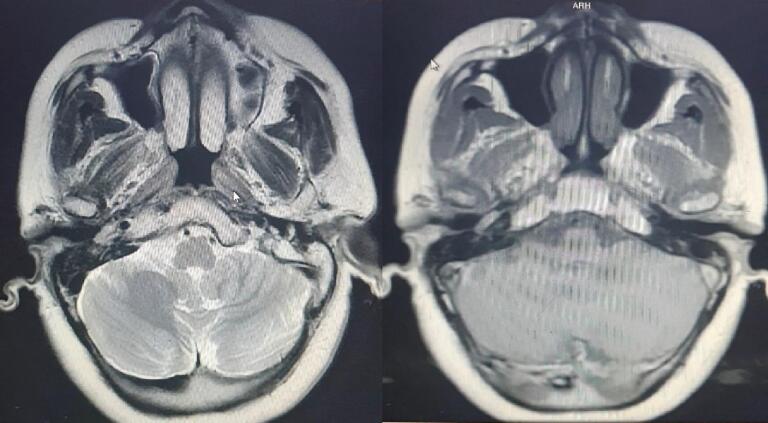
A MRI showing a lesion nearness the cochlea with high signal on T2, clear borders and good enhance.
